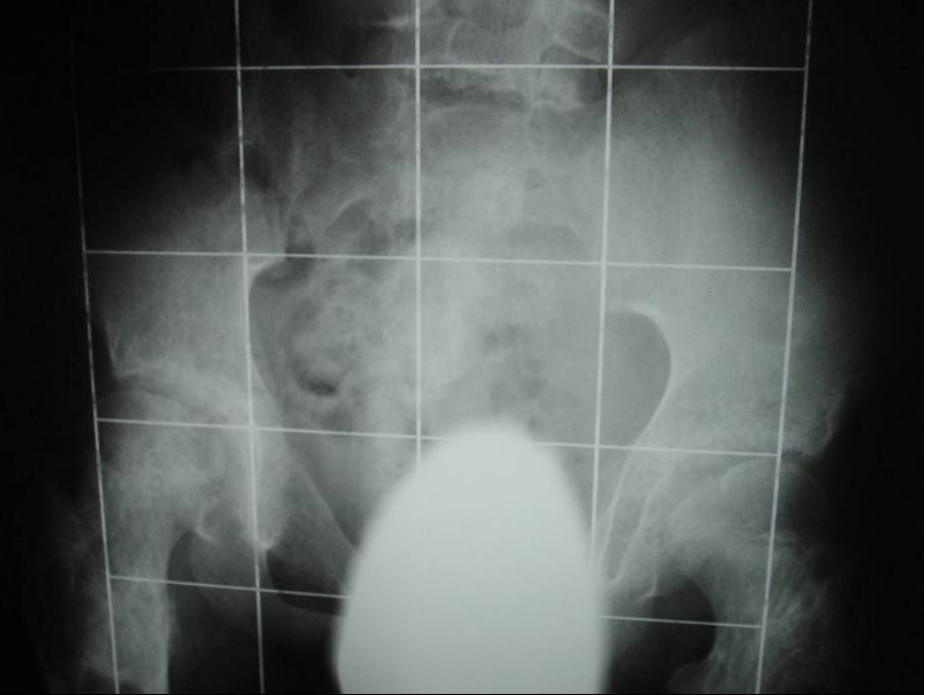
Anteroposterior pelvic radiograph showed coxa valga associated with defective modeling of the femoral necks and extensive striae of fibro-chondromatosis type.
